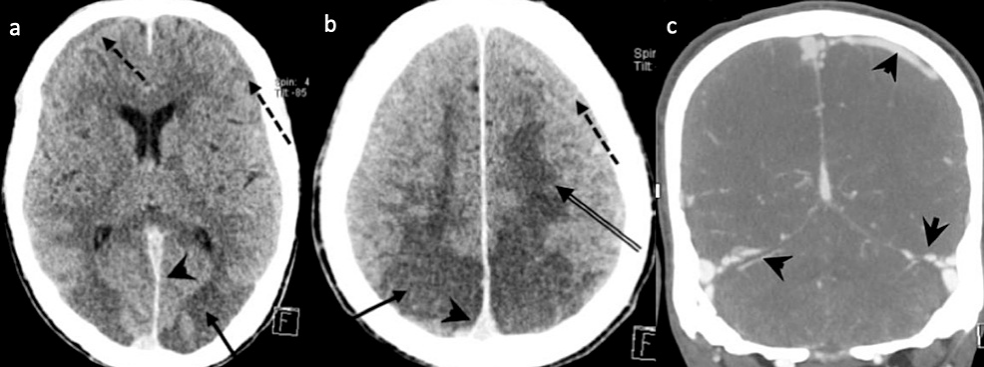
Non-enhanced CT of the brain. (a, b) Bilateral symmetrical white matter hypodense changes (vasogenic edema) involving parieto-occipital lobes (arrows) and bifrontal regions “superior frontal sulcus pattern” (double arrows), suggestive of posterior reversible encephalopathy syndrome (PRES). There were hyperdense-engorged dural venous sinuses (arrowheads) and bilateral subdural effusion (dashed arrows) as sequelae of intracranial hypotension. (c) CT venogram showing engorged dural venous sinuses and cortical veins (arrowheads).
